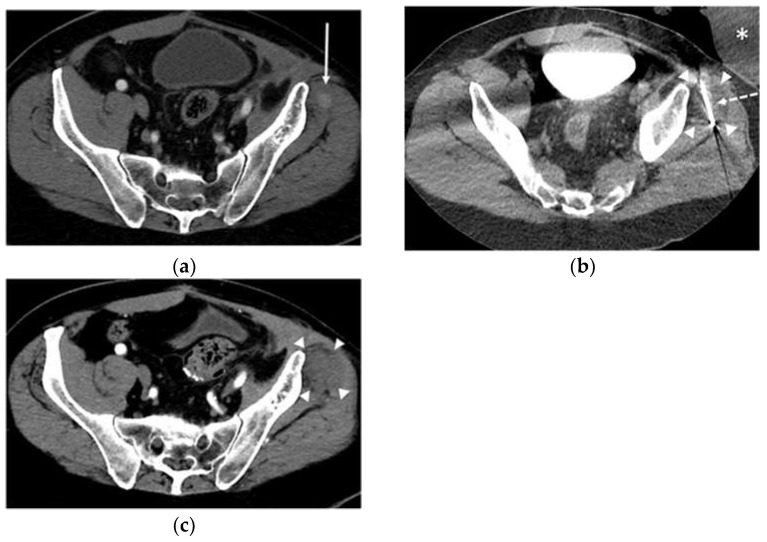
A 61-year-old male with metastatic high-grade undifferentiated pleomorphic sarcoma of left gluteus medius muscle: (a) Contrast-enhanced axial CT image shows a 1.2 cm enhancing soft tissue mass (arrow) in the left gluteus medius muscle compatible with distant metastatic disease. (b) Axial CT image acquired at 5 min into the freeze cycle during cryoablation procedure shows one of the three probes (dashed arrow) located across the mass and developing ice ball (arrowheads). Warm-saline bag (asterisk) was placed over the skin for protection during cryoablation. (c) Contrast-enhanced axial CT image 3 months after cryoablation demonstrated post-ablation changes of the left gluteus medius muscle (arrowheads) without residual or recurrent disease. CT, computed tomography.
